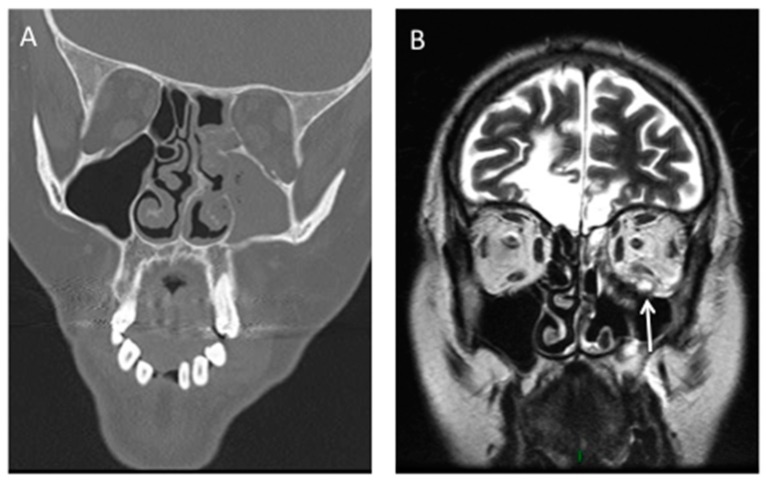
Rhino-Orbito-Cerebral mucormycosis in a diabetic patient. (A): CT scan shows left maxillary, ethmoidal and sphenoidal sinusitis complicated by osteolysis of the maxillary sinus. (B): Coronal T2-weighted MR image shows intra-orbital mass (arrow) compatible with fungal extension.
